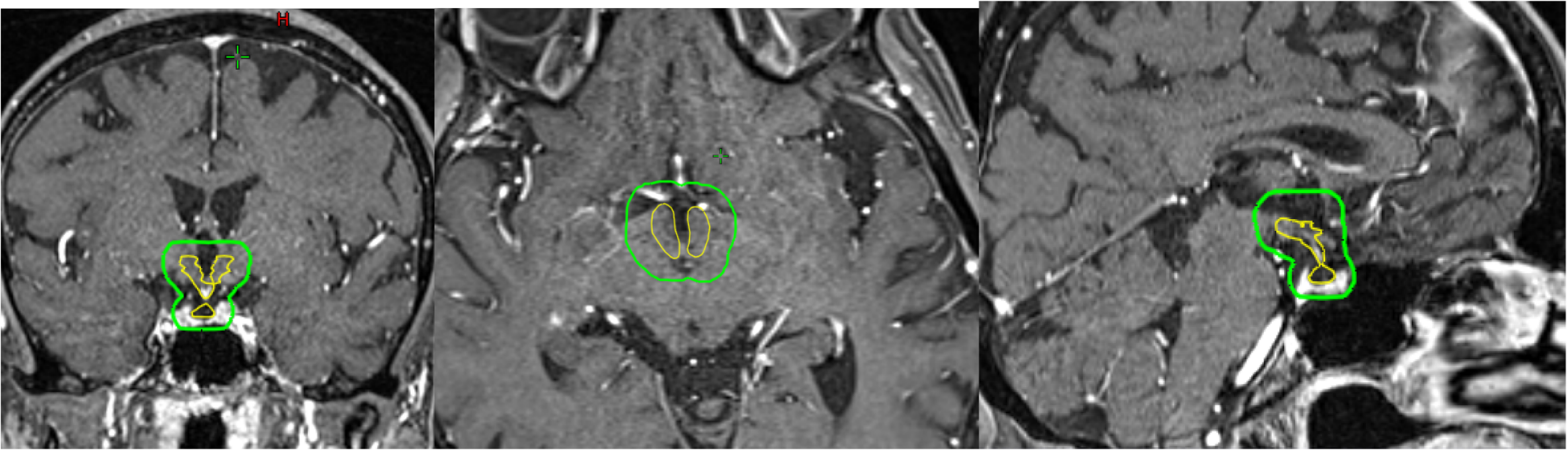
Contouring example of the HT-P axis (gadolinium enhanced T1 MRI)
